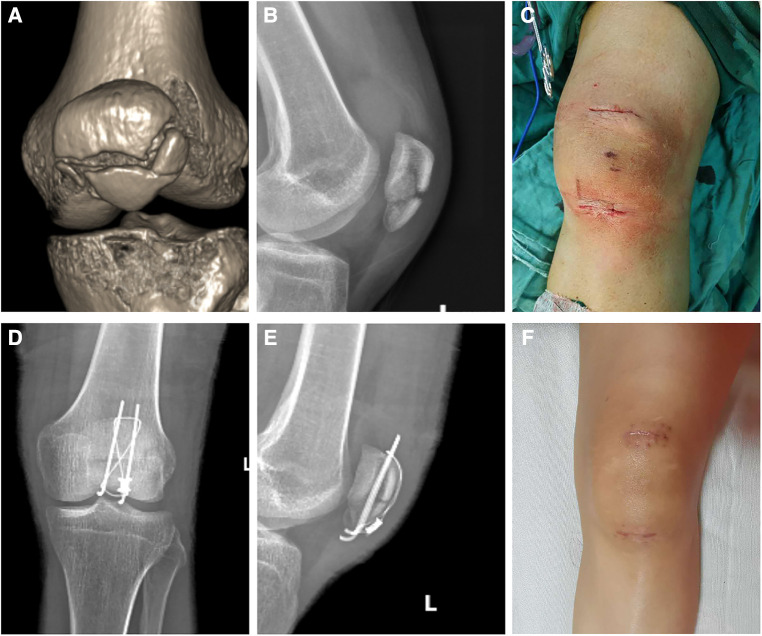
A 32-year-old female patient suffered patellar fracture in a traffic accident and was operated by using the minimally invasive technique. (A) 3D reconstruction image of patellar computer tomography scanning shows the patellar fracture. (B) Lateral view of a preoperative radiograph of the patella. (C) Photograph of an incision taken immediately after surgery. (D) Anteroposterior view of a postoperative radiograph of the knee joint. (E) Lateral view of the same radiograph shows anatomic reduction. (F) Photograph of an incision taken in the fourth month after surgery.
